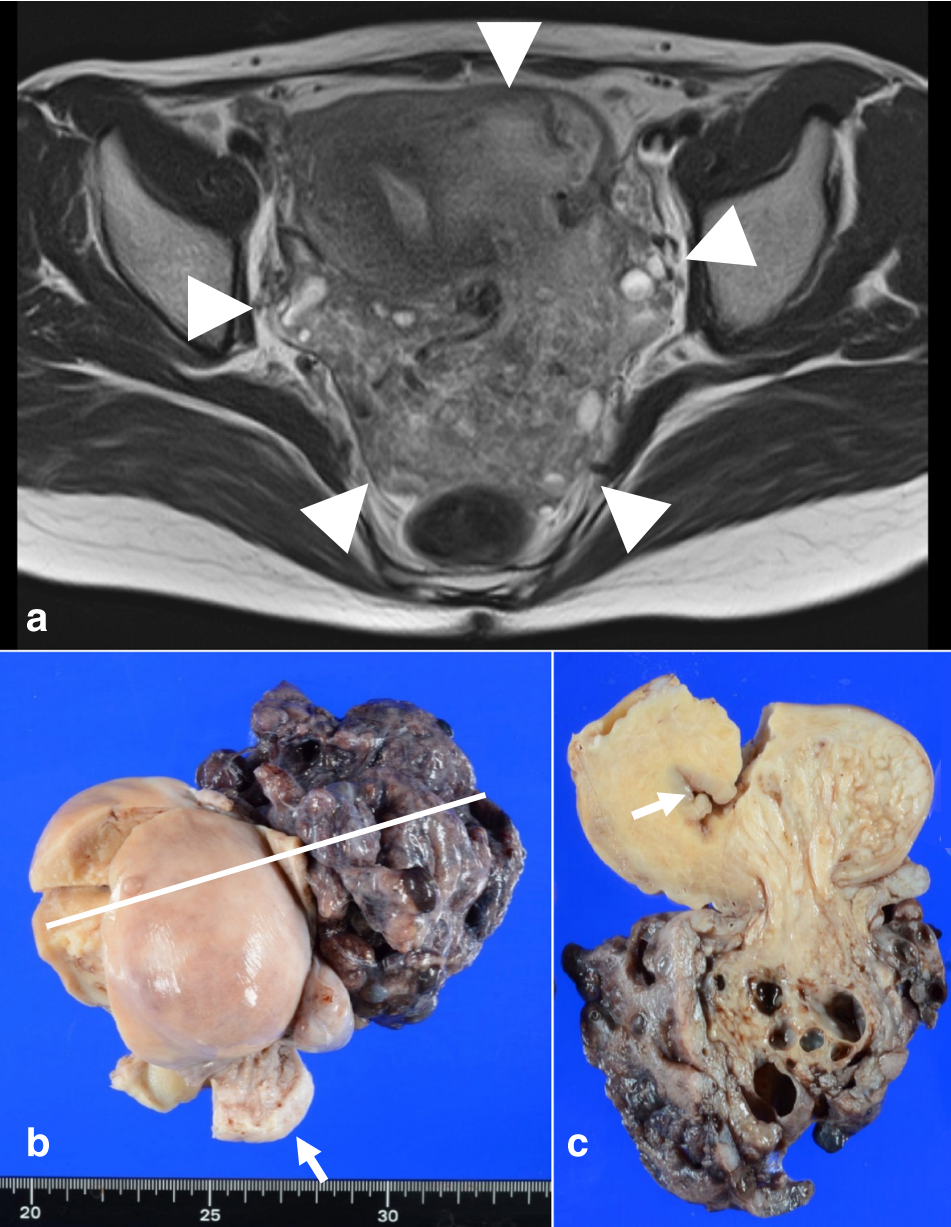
Magnetic resonance imaging and gross appearance of the tumor. a Axial T2-weighted image shows a pelvic mass, measuring 10 × 9 × 7 cm, located in the left posterolateral myometrial wall, with extrauterine extension to the pelvic cavity (arrowhead). b Macroscopically, a large exophytic mass protruding from the posterior uterine wall into the pelvic cavity is observed. The mass contains multiple deep red multiple nodules, giving it a placenta-like appearance. Uterine cervix is not affected (arrow). White line demonstrates the cut line shown on Fig. 1c. c The extrauterine mass contains numerous hemorrhagic cysts. The extrauterine mass shows continuity as a white indistinct multinodular mass in the myometrium. Endometrium is preserved (arrow)
